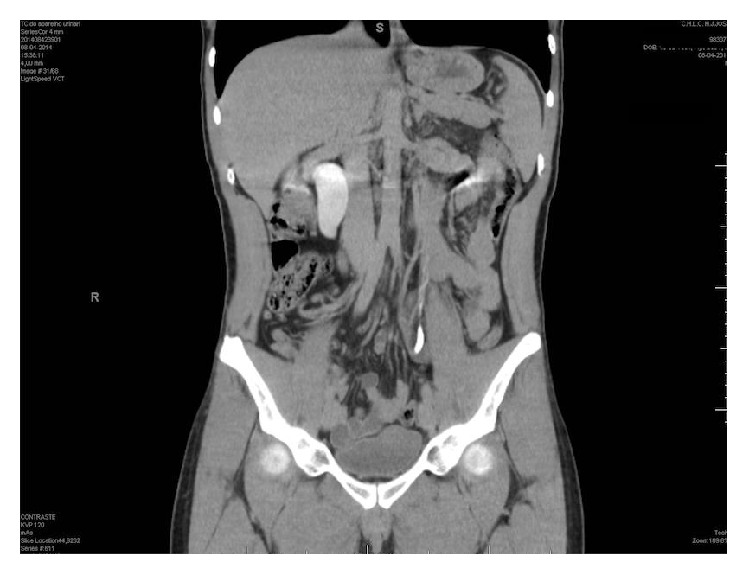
CT scan image showing the “reverse J” deformity.
